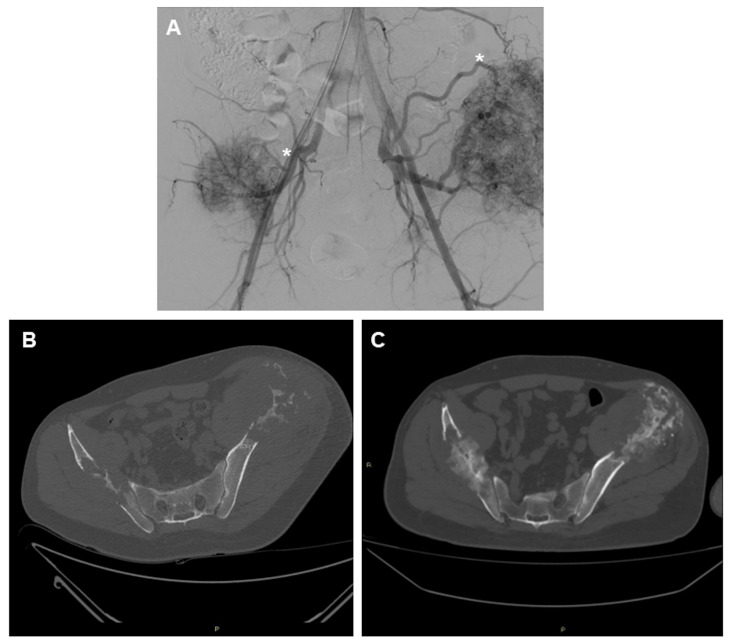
A 56-year-old male patient with two large metastases from renal cancer localized in both iliac bones. (A) panoramic DSA angiogram showing two large hypervascular masses supplied by hypertrophic vessels arising mainly from in internal iliac artery (asterisk). (B) Pre-embolization CT-scan demonstrating the two large lytic lesions of both iliac bones and (C) 6-month follow-up CT showing marked calcification of the lesions.
